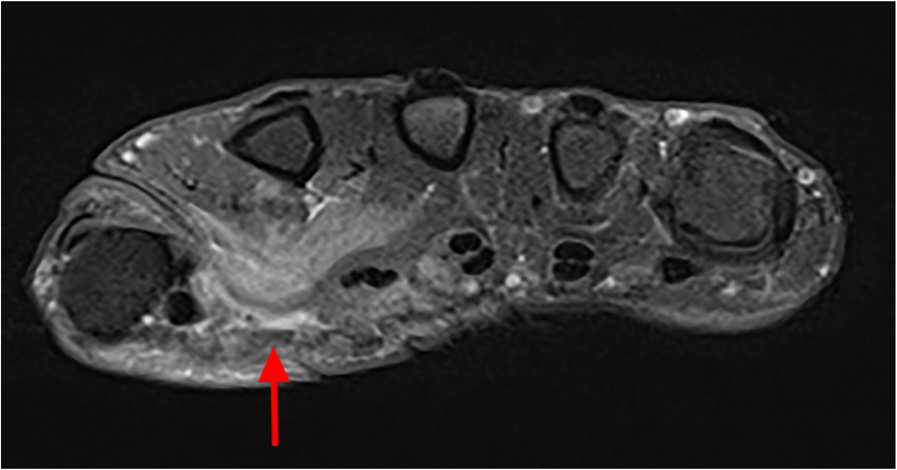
A magnetic resonance imaging examination of his right hand at initial presentation. T1-weighted fat-saturated axial magnetic resonance image shows diffuse enhancement of the muscles and the palmar aponeurosis (*arrow*). There are no pathological features in his bones or joints
